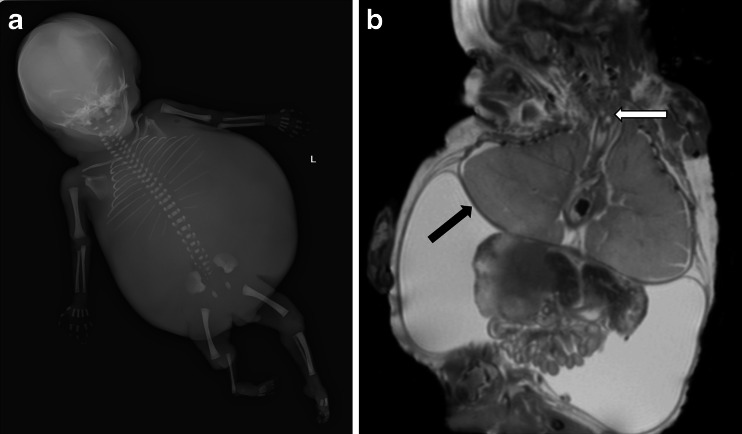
Case 2. Postmortem radiography and MRI in a male foetus with congenital high airway obstruction syndrome who was terminated at 24 weeks’ gestation. a Postmortem anteroposterior skeletal radiograph shows gross soft-tissue swelling of the thorax and abdomen, with splaying of the ribcage and right fibular hemimelia and severe talipes equinovarus. b Coronal high-resolution T2-weighted MRI 6 days after death shows similar features of disproportionately gross ascites, marked distension of both lungs with eversion of the diaphragm (*black arrow*) and a centrally placed heart. Even on sagittal high-resolution T2-weighted MR images (not shown) it was difficult to determine the level of the airway obstruction (*white arrow* in b)
